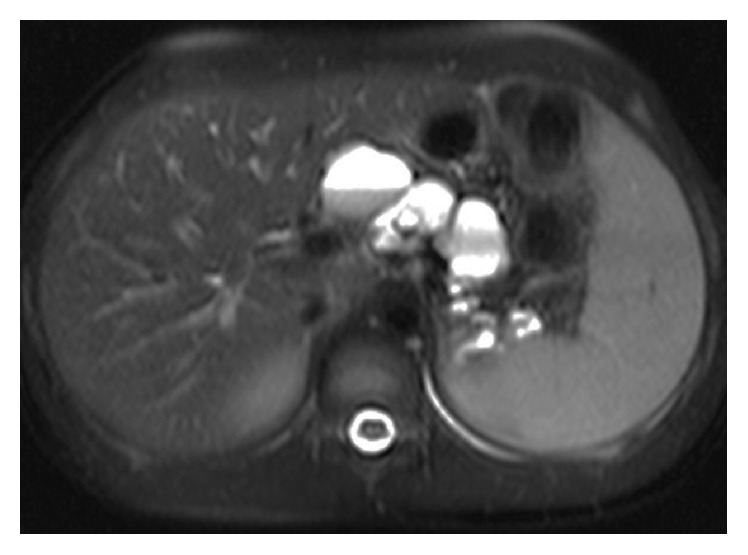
T2-weighted fat-saturated transverse MR image also shows multiple high signal, cystic lesions of various size at the expected region of the body of the pancreas. Note the fluid-fluid level in the largest cyst.
